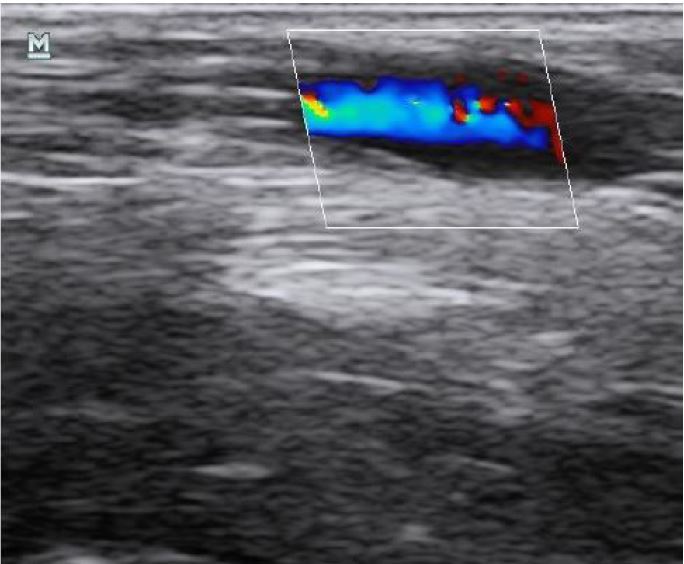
Positive “saline flush test” with color flow Doppler.
